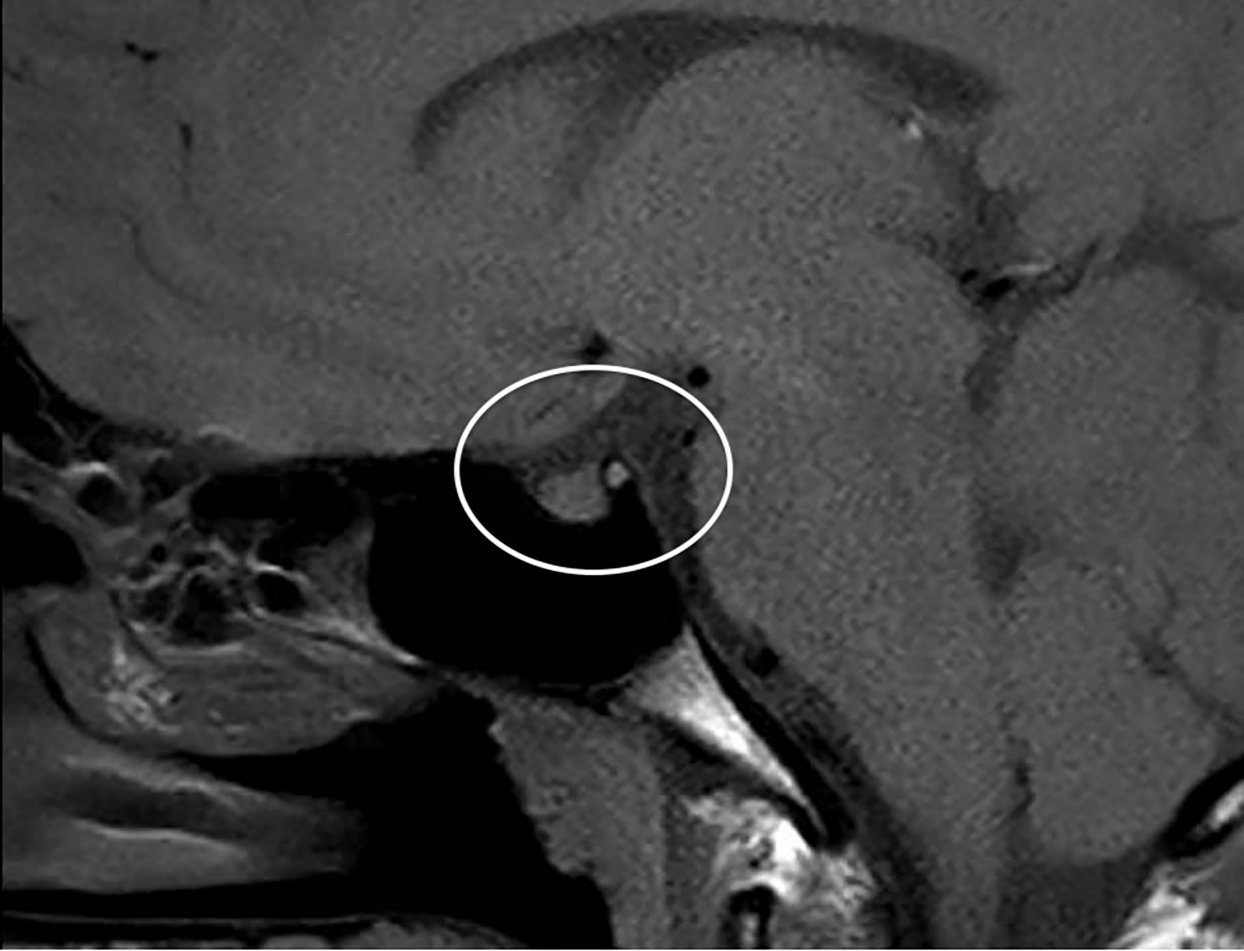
Brain MRI after 2 months: stable thickened pituitary stalk and undetectable posterior pituitary (circle).
